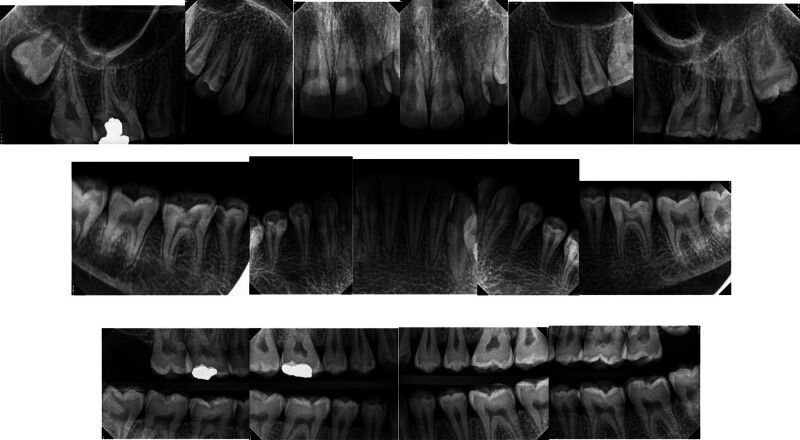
Preoperative full-mouth x-rays.
